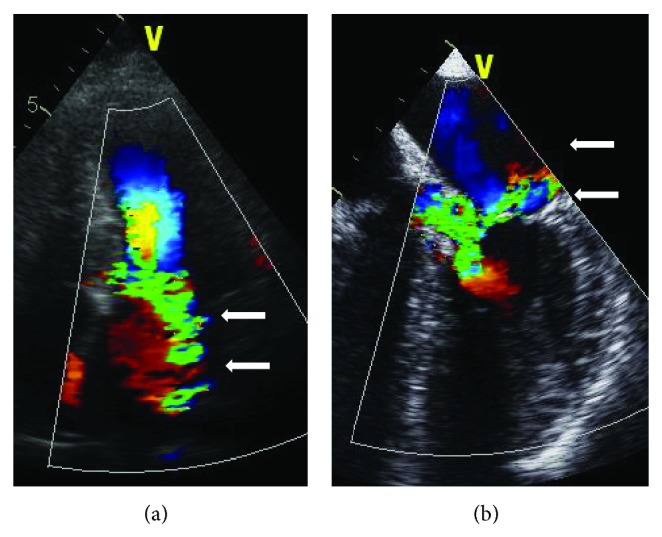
Typical mitral regurgitation associated with SAM and subaortic LVOT obstruction with a posterolateral jet orientation (arrows) in a transthoracic (a) and transesophageal view (b). LA: left atrium; RA: right atrium; LV: left ventricle; Ao: aorta; and IVS: interventricular septum.
